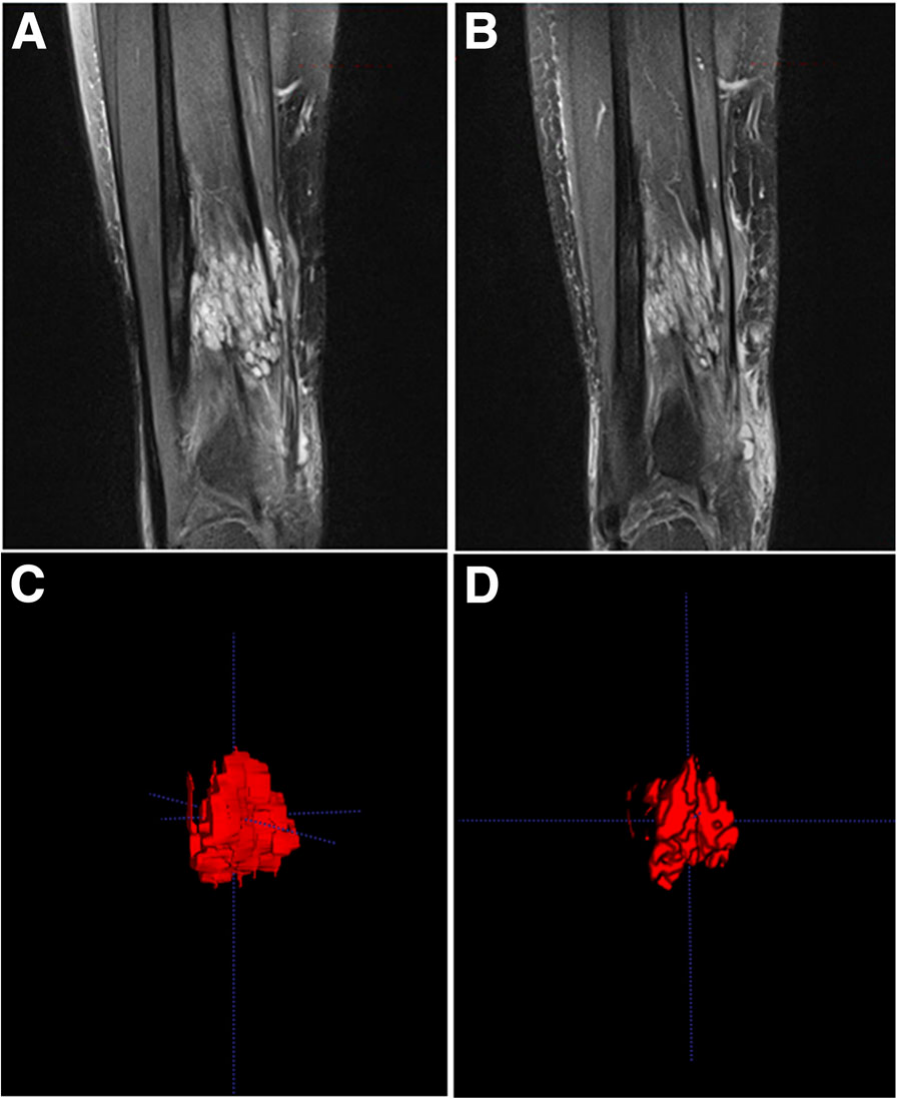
Classical MRI sequences and 3D-volumes of the malformation (patient #15) before initiation of sirolimus (a, c) and after 12 months of treatment (b, d). Notice volume reduction from 14 cm^3^ to 12,3 cm^3^
